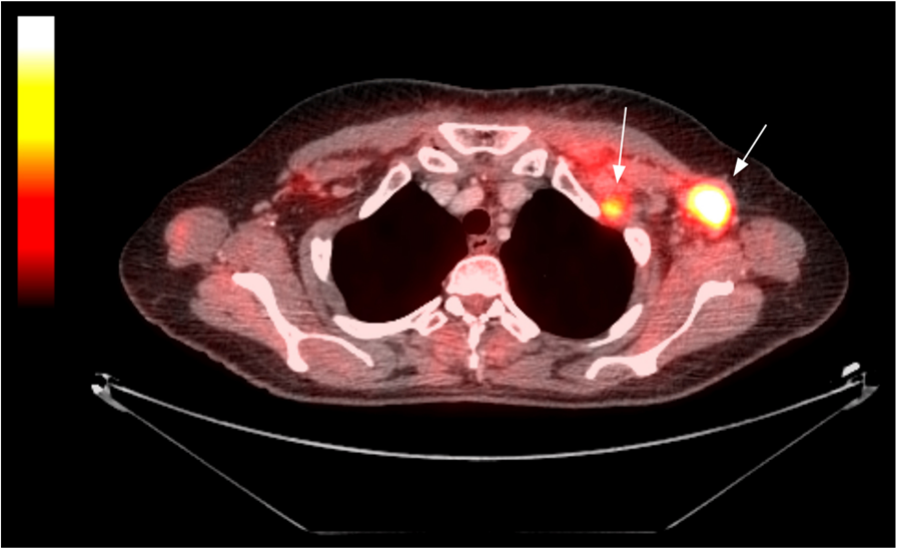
PET/CT showing multiple FDG-avid lymph nodes in the left axilla and under the pectoralis major muscle
